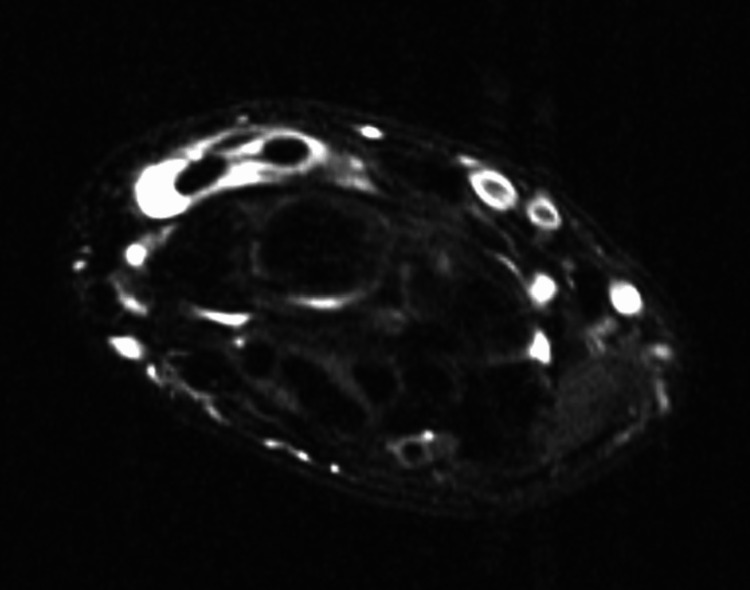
Axial T2-weighted images of the patient’s right wrist demonstrating fluid within the tendon sheaths of the ECRB/ECRL and EPL ECRB: Extensor carpi radialis brevis; ECRL: Extensor carpi radialis longus; EPL: Extensor pollicis longus
